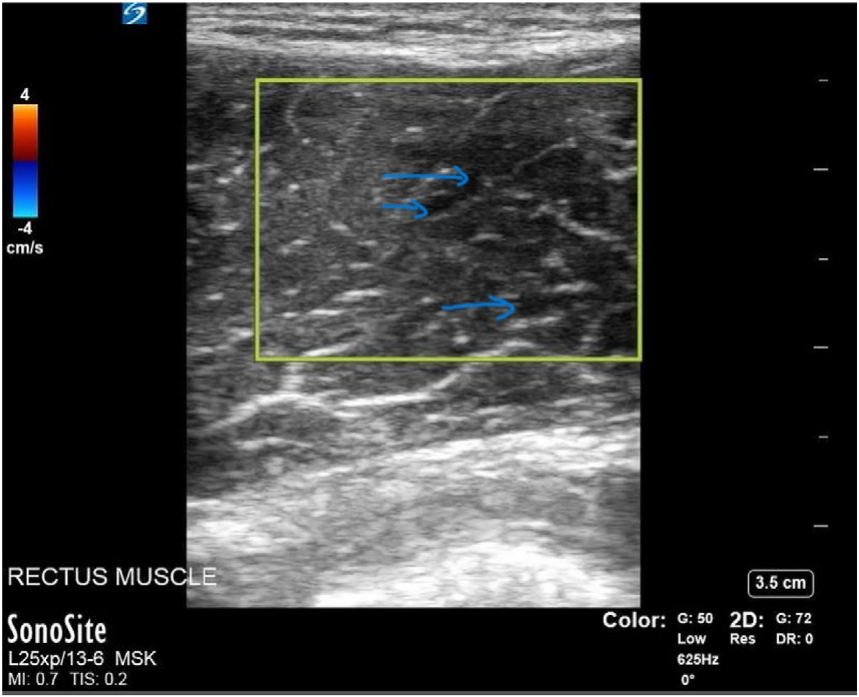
Point-of-care ultrasound of the right rectus abdominus muscle in the short-axis view demonstrating muscle fiber elongation with intramuscular edema indicated by the blue arrows. Again, the yellow rectangle demonstrates no evidence of small vascular structures.
